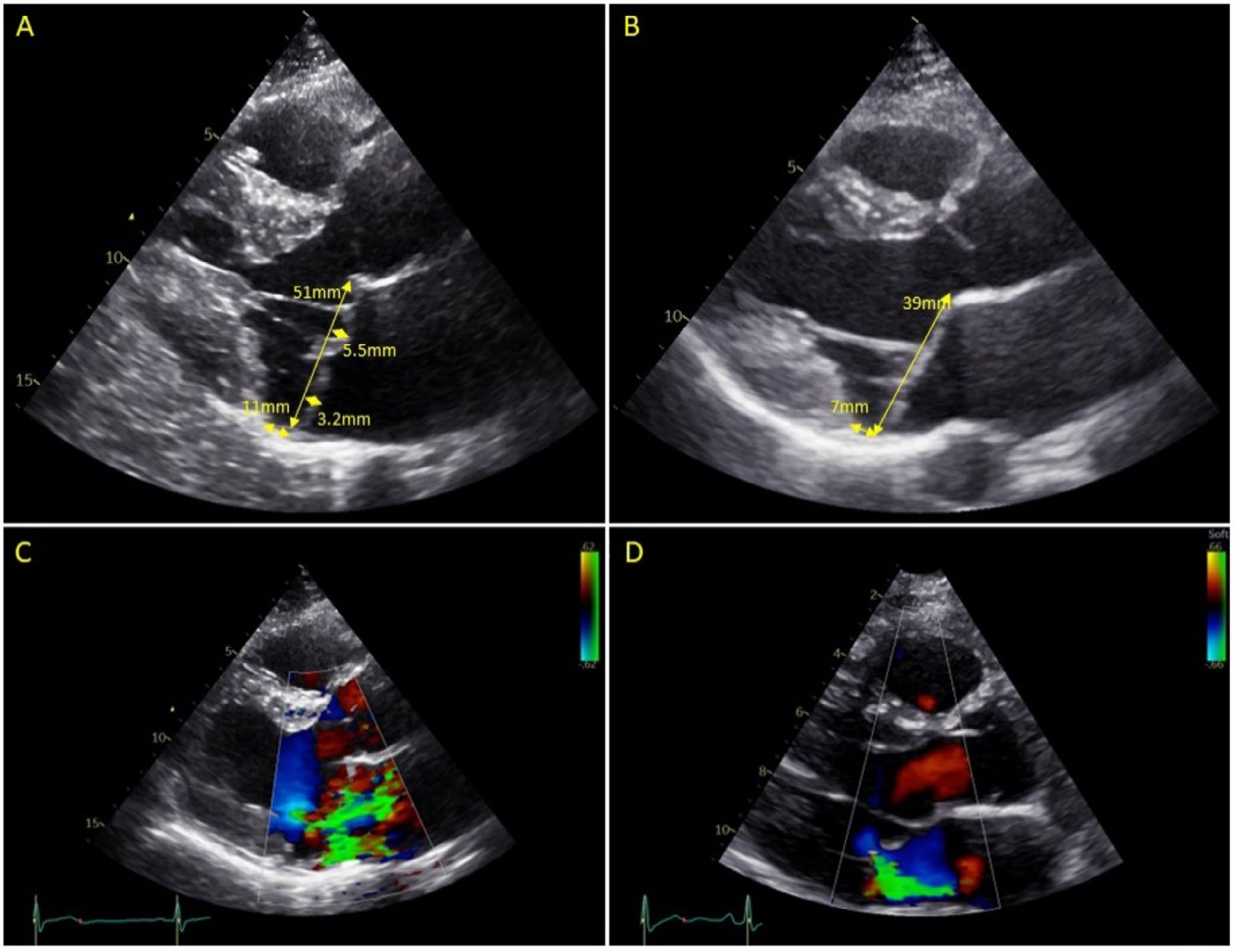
Echocardiograms of the family. (A) Echocardiogram of the proband (III.1) with Barlow's disease showing bi‐leaflet prolapse (> 2 mm), annular dilatation (51 mm) and mitral annular disjunction (11 mm) and (B) echocardiogram of the sister (III.2) with bi‐leaflet billowing, annular dilatation (39 mm) and mitral annular disjunction (7 mm). (C) Color echocardiogram of the proband showing severe mitral regurgitation and (D) color echocardiogram of the sister showing mild mitral regurgitation.
